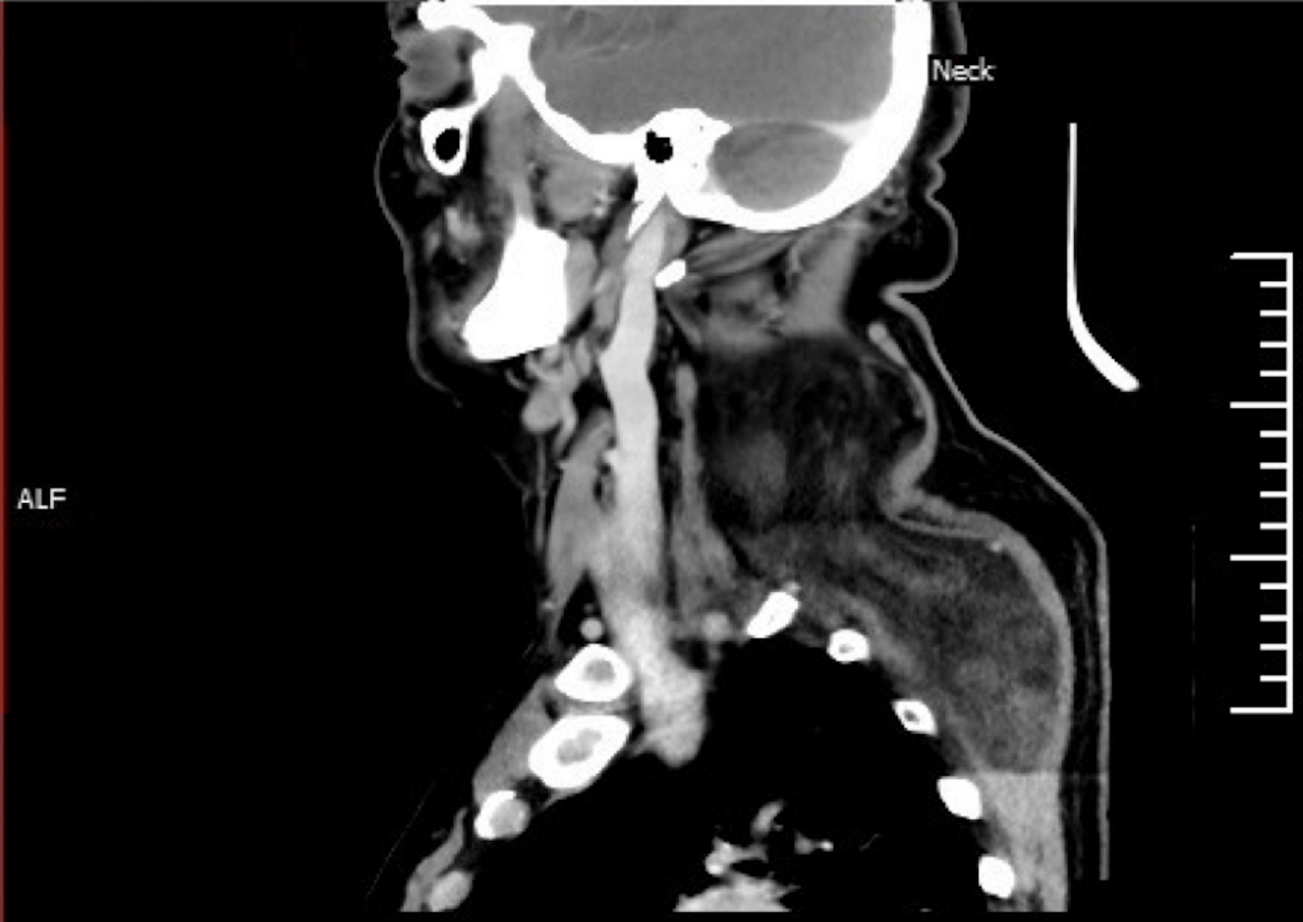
A sagittal view of the neck CT scan shows a huge cervical mass involving the posterior triangle and extending to the back.
